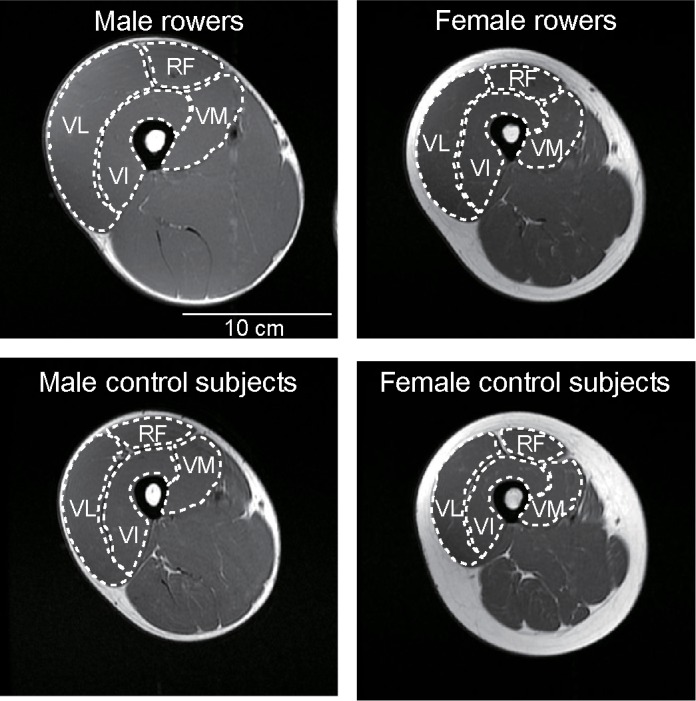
Examples of T1-weighted magnetic resonance images of the mid-thigh. The outline of individual muscles of the quadriceps is indicated by white dotted lines. VM, vastus medialis; VL, vastus lateralis; VI, vastus intermedius; RF, rectus femoris.
